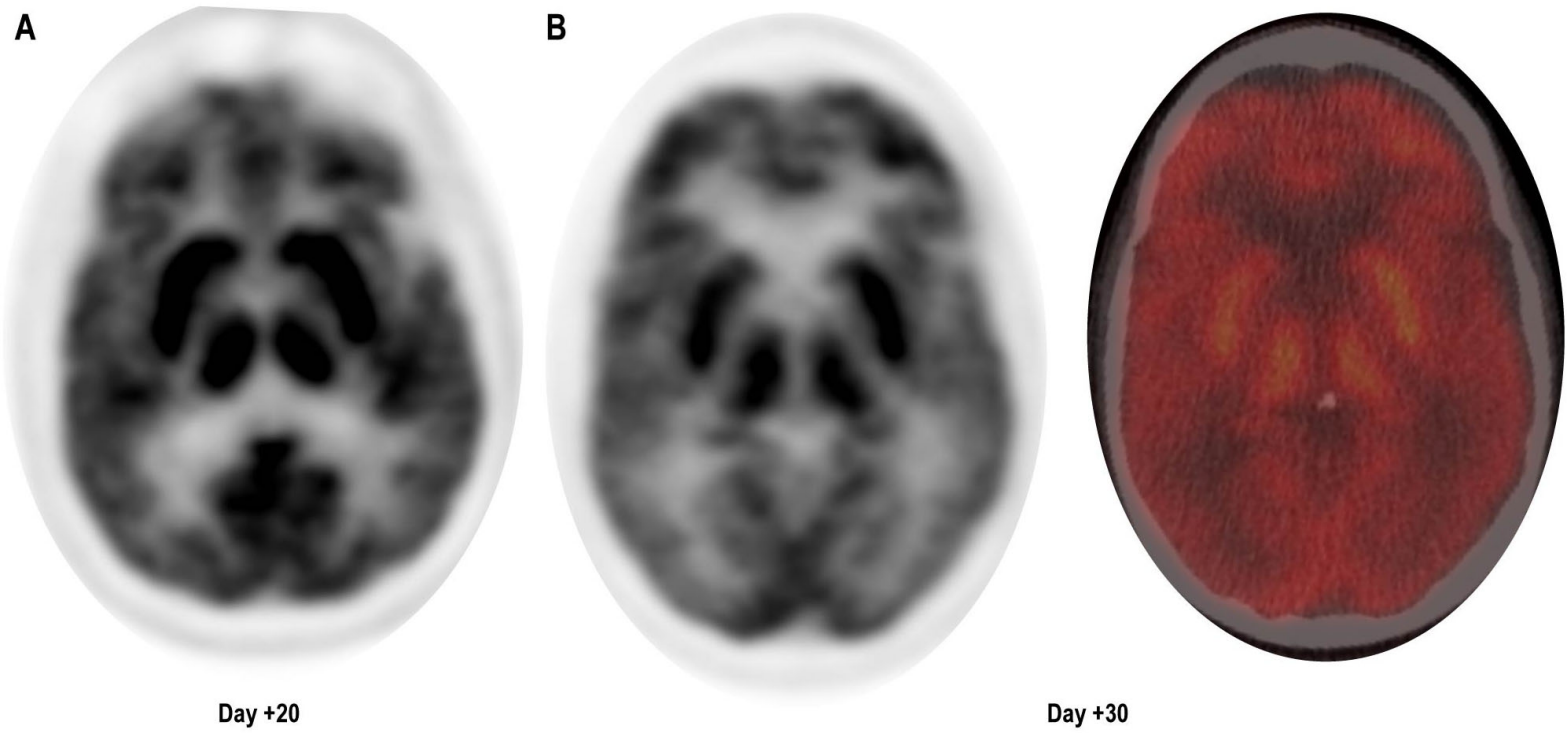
Brain (18) F-fluorodeoxyglucose (FDG) positron emission tomography (PET) CT demonstrating diffuse symmetrically enhanced tracer uptake in the caudate, putamen, and thalamus. (A) Day + 20, unfused PET. (B) Day +30, unfused PET (left) and fused color image (right).
